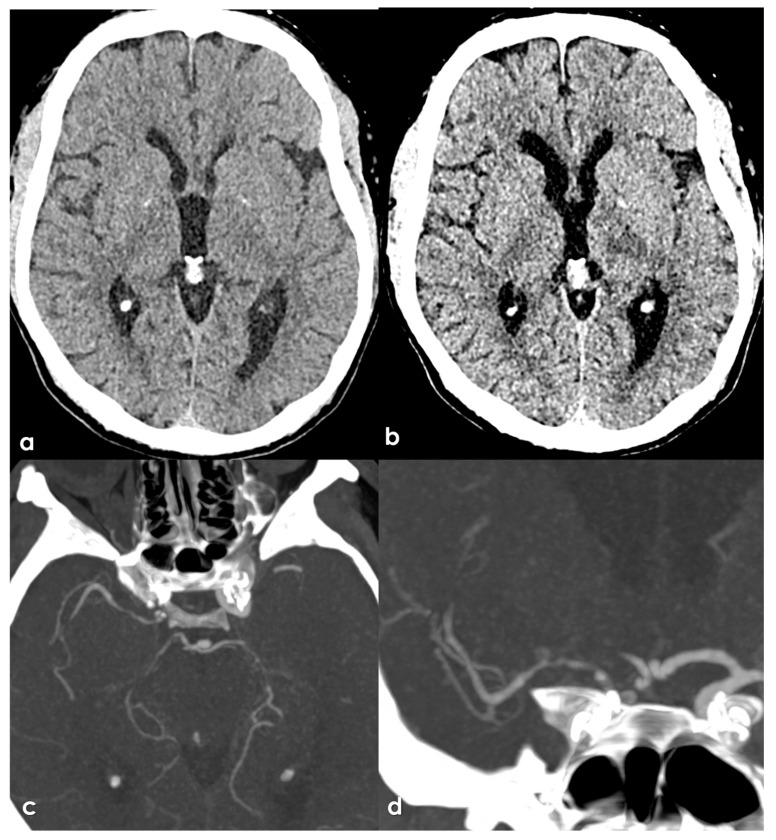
CT-based study of a patient with PAD in the left posterior cerebral artery (PCA) territory and multifocal intracranial stenosis. Panel (a,b) show non contrast CT scan at the baseline (panel (a)) and after 24 h (panel (b)), when a recent left capsular ischemic lesion appears. Panels (c,d) show CTA reconstructed in the axial and coronal plane, respectively, using the MIP/MPR protocol with bilateral ICA stenosis and severe left P2 PCA stenosis.
